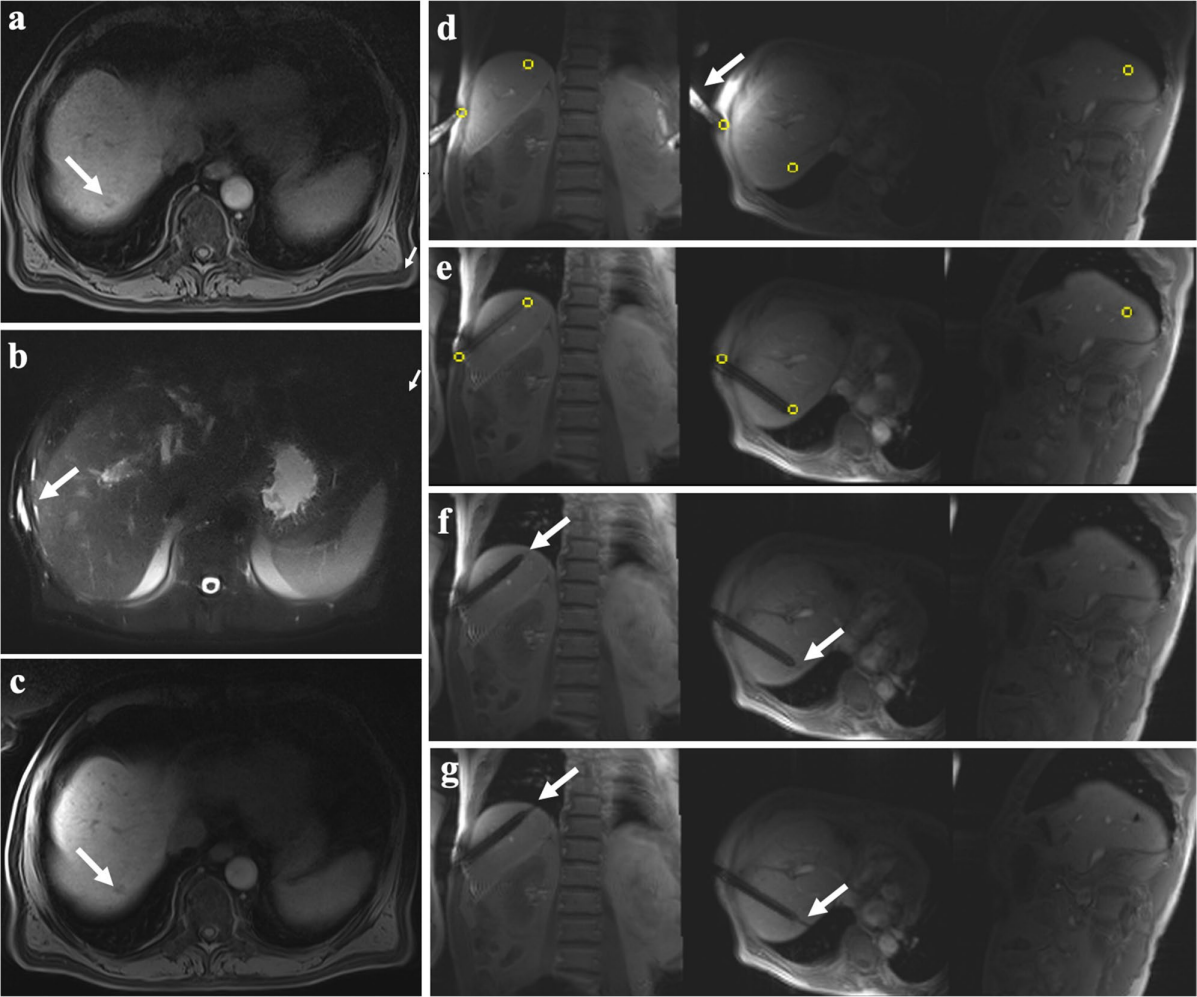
A 67-year-old male patient with known HCC and new suspected FLL located in liver segment 8 undergoing MRI-guided liver biopsy with an intercostal approach. a Preprocedural axial T1w GRE sequence after liver-specific contrast agent application revealed a small target lesion at the hepatic dome with a diameter of 7 mm. d Defining and digital marking of entry point and target lesion (yellow tags) for procedure planning in use of three-dimensional real-time T1w GRE sequences and finger-pointing (arrow). e Three-dimensional real-time T1w GRE sequences confirmed guidance and angulation of 16-gauge coaxial needle while interactive graphical modification of the slice geometry. f After fading out the yellow tags, the three-dimensional real-time T1w GRE sequences revealed the exact position of the needle tip at the lesion border (arrows). g Firing of the biopsy system under real-time monitoring of three-dimensional T1w GRE sequences. b Postprocedural axial T2w fat-saturated TSE sequences excluded relevant bleeding or subcapsular hematoma. c Postprocedural axial T1w GRE sequences showed two thin hypodense lines within the small FLL, compatible with matching puncture tracts
